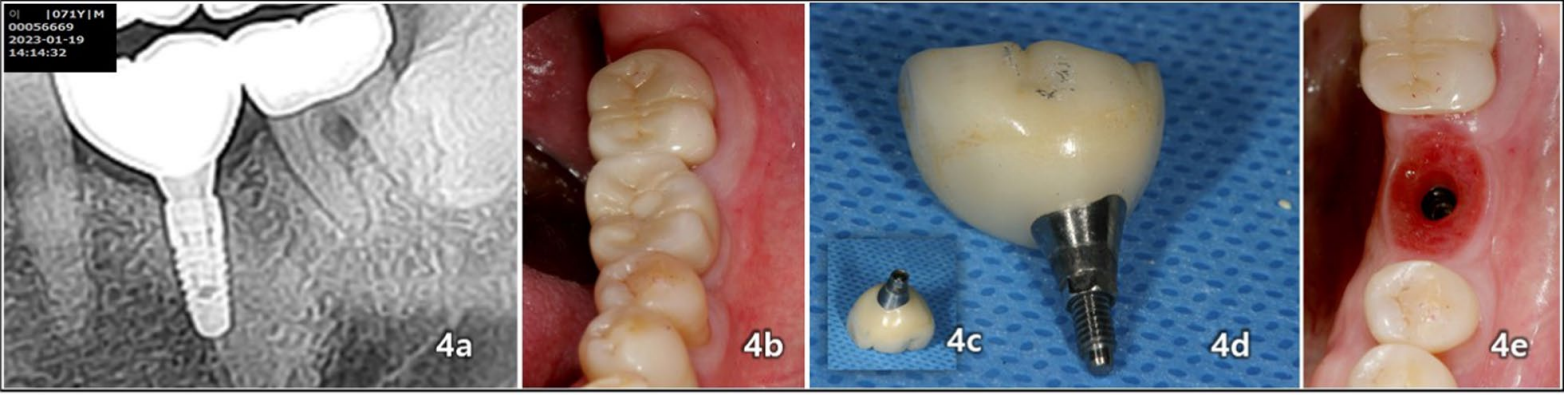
A 10-year clinical follow-up after immediate functional loading of the implant revealed the following: a A 10-year follow-up involving the periapical radiographic image on the operation site taken on January 19, 2023. b An intraoral occlusal view of implant-fixed screw-fastening Monolithic Zirconia crown on the lower left first molar site. c, d, e Examination of the crown and mucosae of the transmucosal part after removal of the definitive Zirconia crown on the operation site. Notably, there was an absence of plaque or debris, and no signs of an inflammatory response were observed
